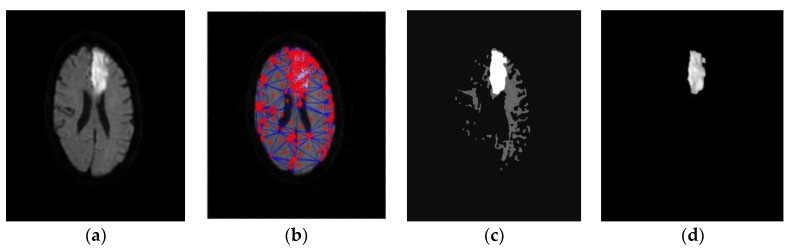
Ischemic stroke lesion is seen on the original DWI (a), Voronoi cells are generated (b), and Delaunay triangulation applied (c), The extracted lesion detected using Delaunay triangulation FODPSO (d) [114].
